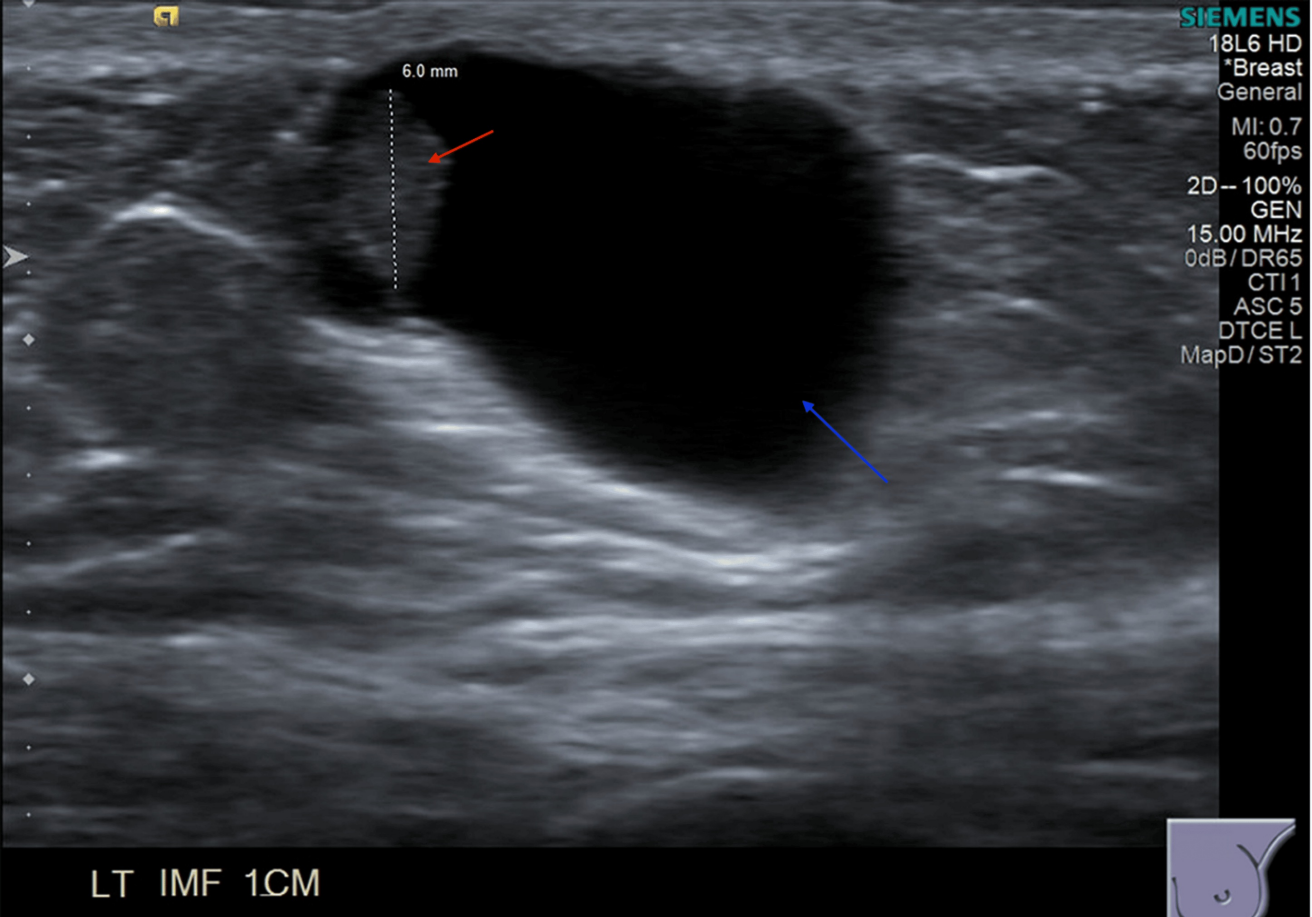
Diagnostic ultrasound image of left inframammary lesion depicting a 1.9 x 1.3 x 1.1 cm cystic lesion (blue arrow) with mural nodule measuring 6 mm (red arrow).
